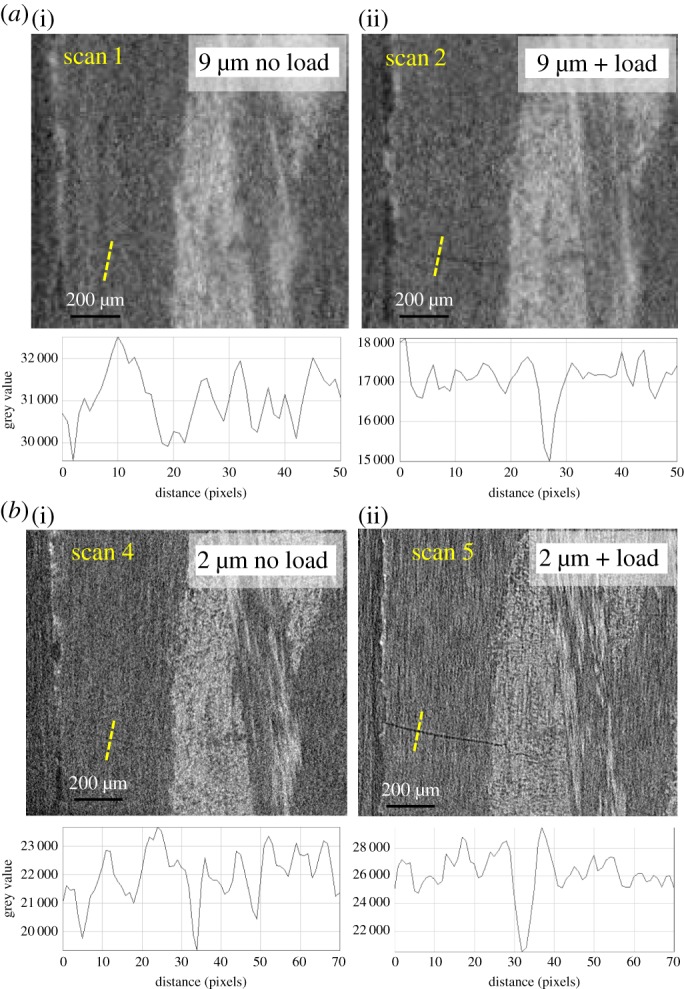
Enlarged virtual CT sections showing the ROI containing crack A. Examining strategies I (increasing the magnification) ((*a*) versus (*b*)) and II (applying a crack opening load) ((*a*(i),*b*(i)) versus (*a*(ii),*b*(ii))). A greyscaleline profile across the location of the crack is shown below each image.
